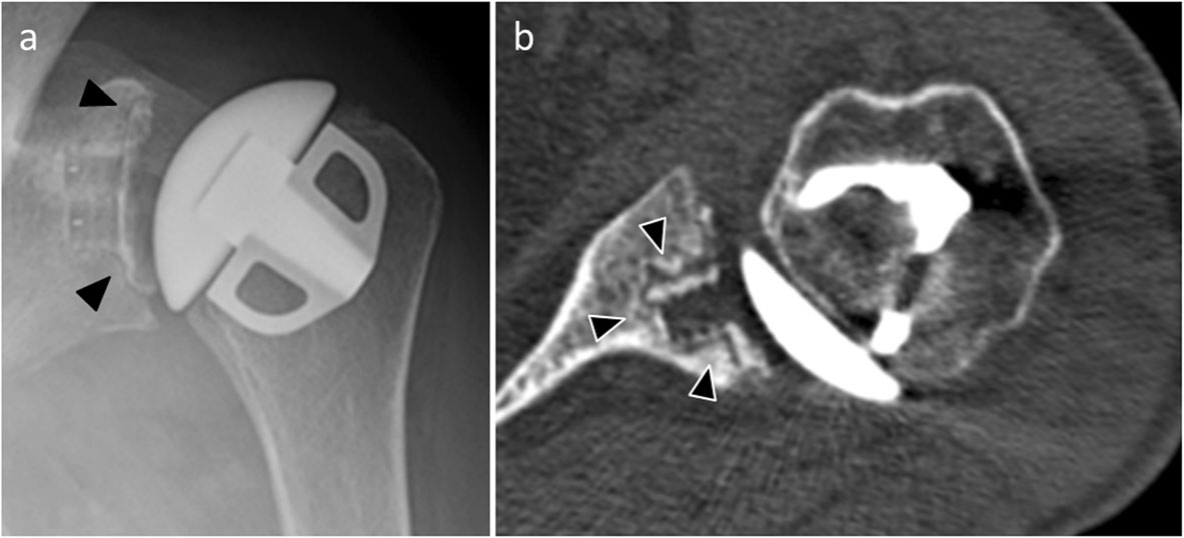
a Anteroposterior shoulder radiograph of a 61-year-old man with total shoulder arthroplasty shows a radiolucent line around the glenoid component (black arrowheads). b Axial CT image in the same patient as a allows a more precise analysis of this radiolucent line (black and white arrowheads).
